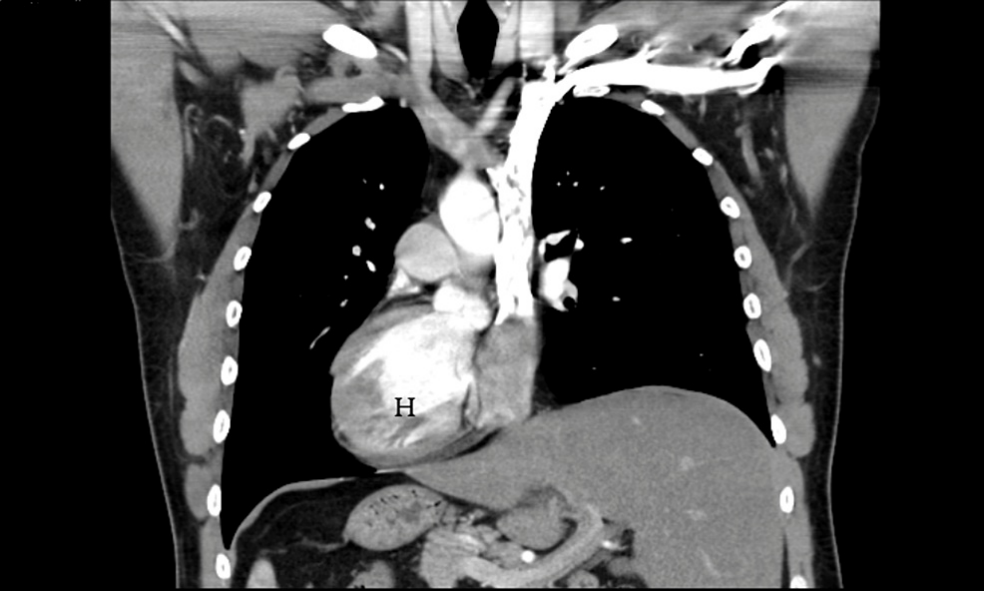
A CT scan of the chest showing the heart (H) lying in the right side (dextrocardia)
